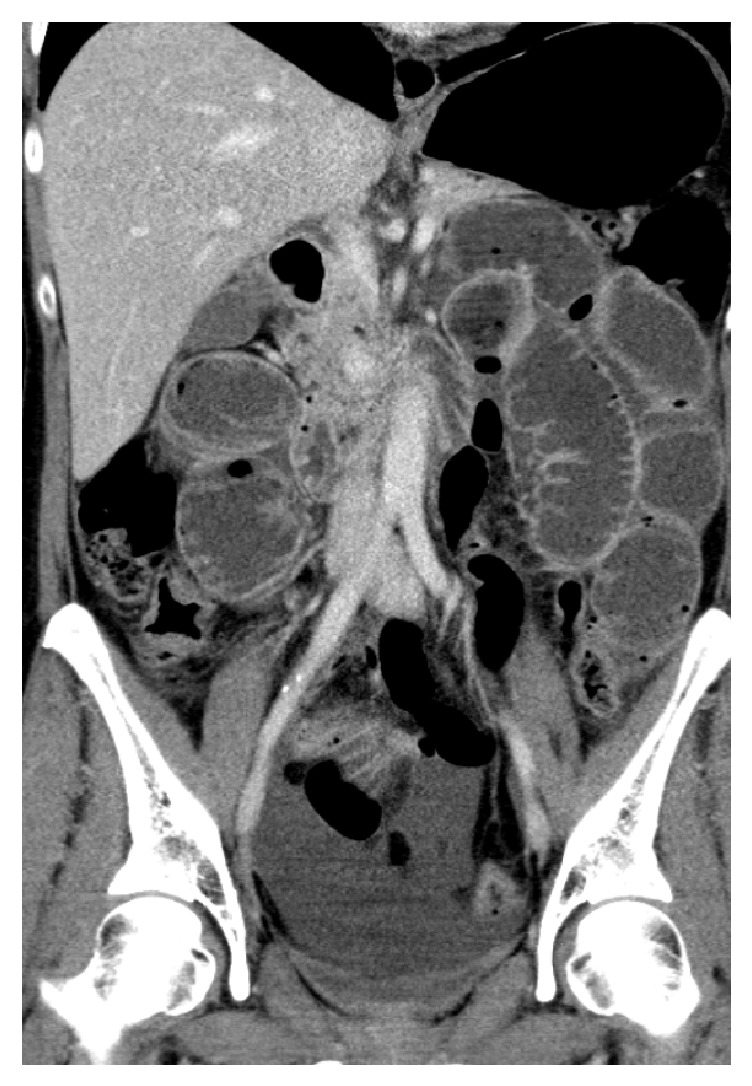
CT of the abdomen: picture of a mechanical small bowel ileus. The colon is not involved. Thus, the caliber jump is most likely in the preterminal ileum, but this cannot be demonstrated on the CT pictures.
